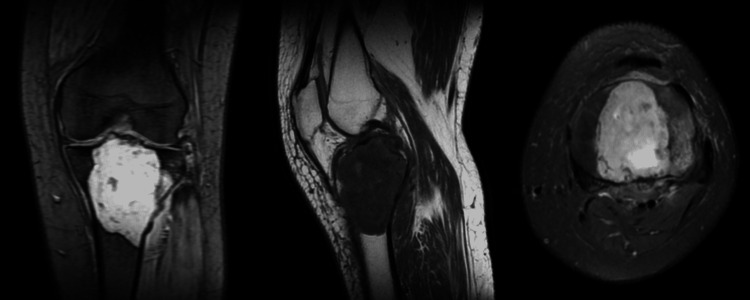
MRI scan suggestive of eccentric soft tissue hyperintense lesion in tibial condyle with peripheral irregular enhancements
